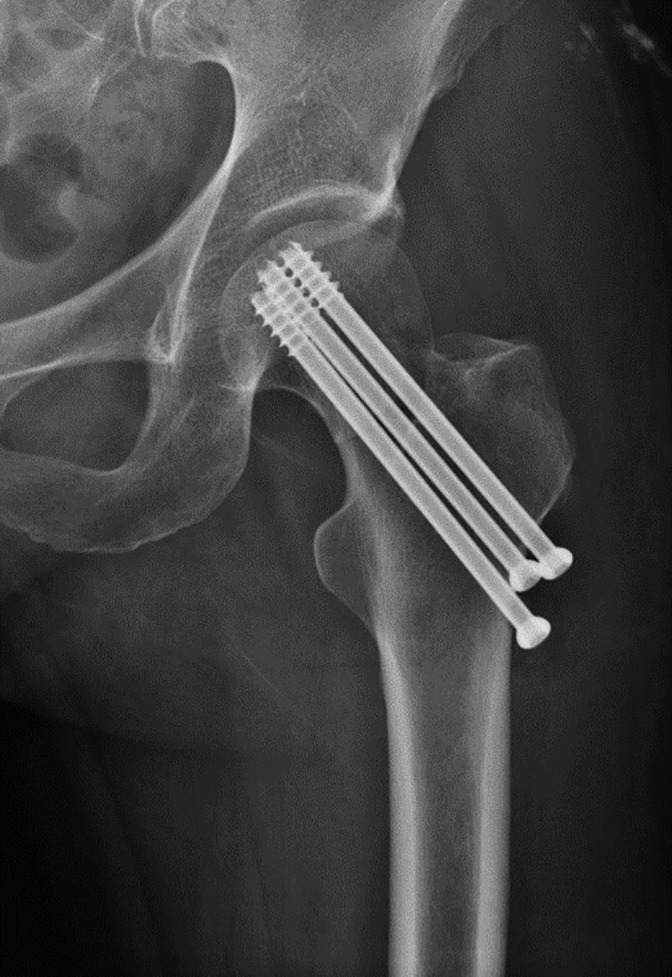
Antero–posterior postoperative radiograph of a right femoral neck fracture in a 58‐year‐old woman.
